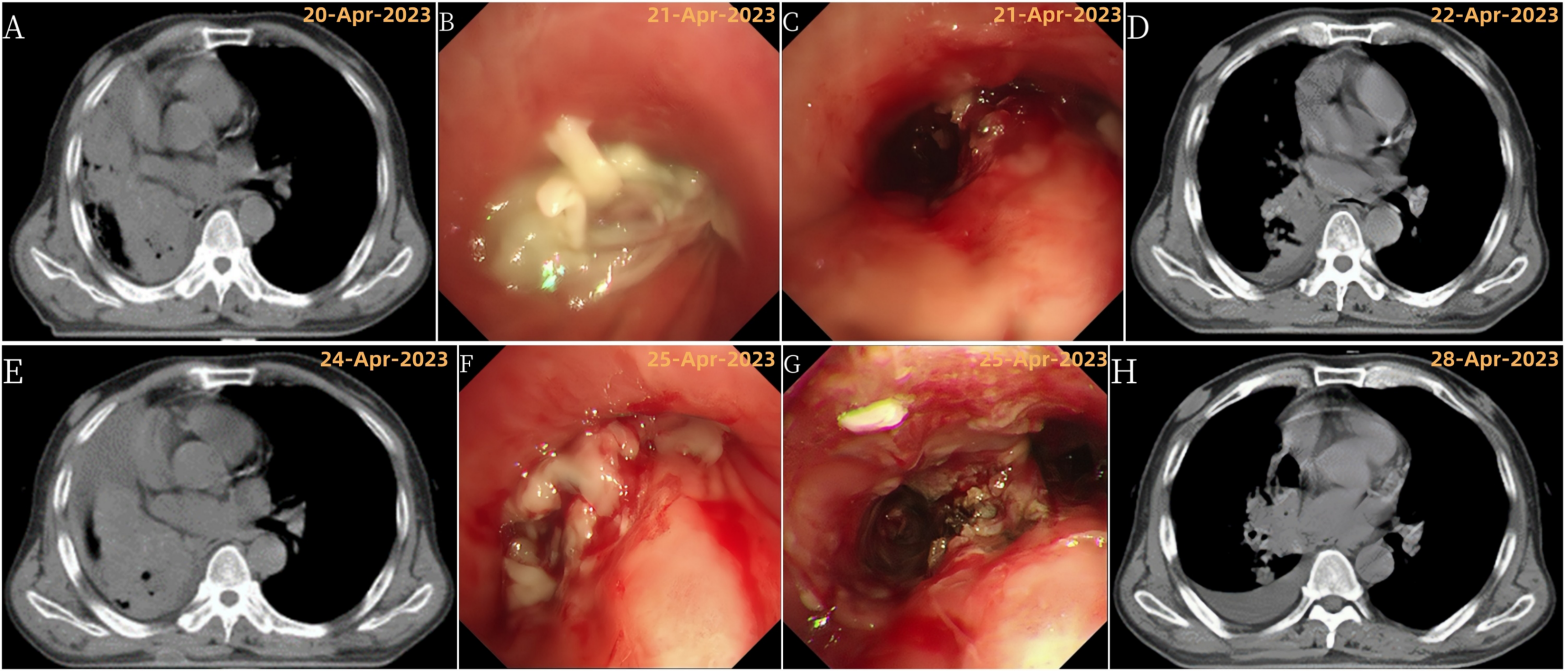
Disease progression and treatment outcomes with multiple bronchoscopic interventions. (A) Chest CT showing right total atelectasis and mediastinal shift to the right; (B) Bronchoscopy showing complete obstruction of the right main bronchus lumen by a new growth; (C) Bronchoscopy after tumor resection showing tumor invasion of the right upper lobe; (D) Chest CT showing improvement in right atelectasis and mediastinal return to normal position after tumor resection; (E) Chest CT showing recurrence of right total atelectasis and right mediastinal displacement; (F) Bronchoscopy showing incomplete obstruction of the right main bronchus, right upper lobe bronchus by a new growth, and occlusion of the right middle bronchus; (G) Bronchoscopy after the third tumor resection; (H) Chest CT showing improvement in right atelectasis and mediastinal return to normal position after tumor resection.
